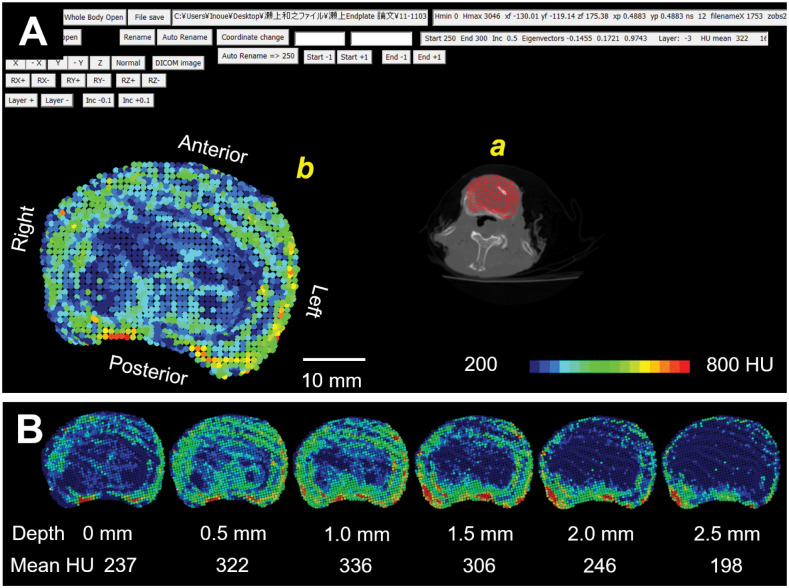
Measurement of Hounsfield Units (HU) across the endplate. A: screenshot of custom-written software for measurement to determine HU values at individual points consisting of a 3D endplate point-cloud model determined by a DICOM dataset. *a;* an axial CT slice (note; only one slice is shown on the screen although multiple slices are involved in the measurement). Red dots indicate location of the endplate point-cloud model. *b;* HU distribution on the endplate point-cloud model. Position and orientation of the point-cloud model can be changed in any increment. B: An example of changes in HU distribution and mean HU values at the surface and at depths 0.5–2.5 mm underneath the endplate surface.
